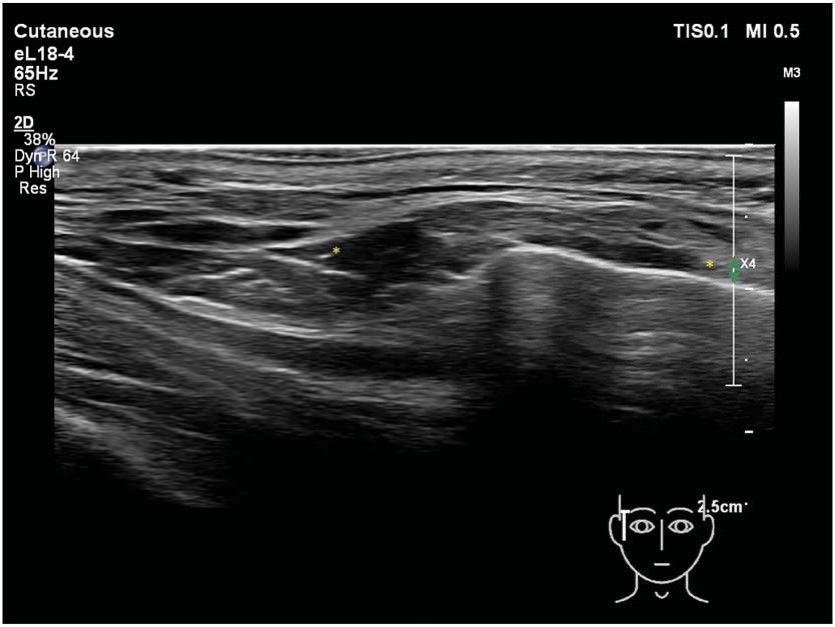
Ultrasound (grayscale, longitudinal view) of the temple and zygomatic area showing filler material injected at the periosteum of the zygomatic bone shifts into the superficial temporal fat pad. Hyaluronic acid filler in between yellow markers (Philips affinity 70 18 MHz).
